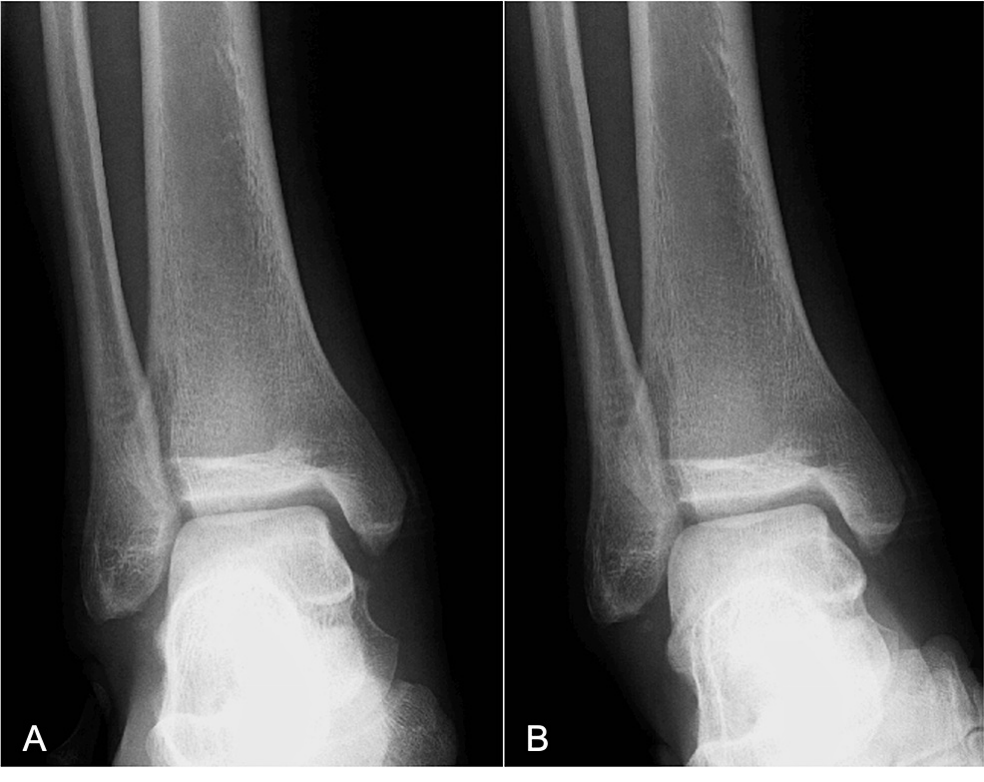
Stress radiography of the right ankle at six weeks postsurgery. The neutral position (A) and inversion stress (B) revealed no ankle instability.
